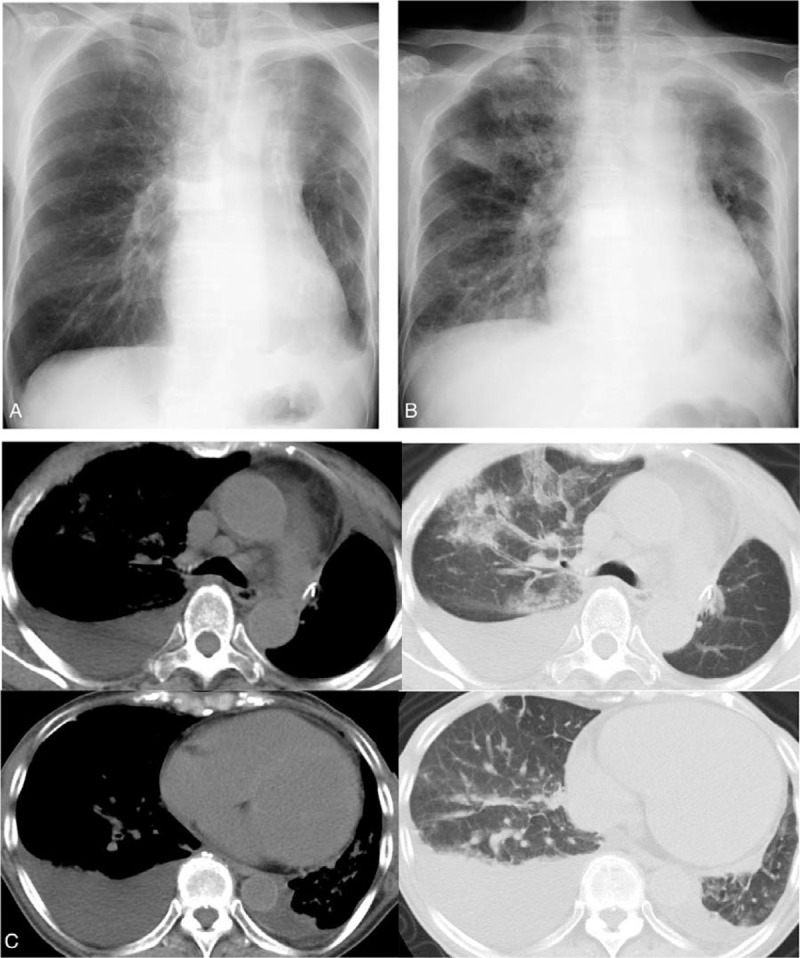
Chest radiography indicates an enlarged cardiac shadow and diffuse ground-glass opacities in both lung fields on admission (A). After osimertinib discontinuation, the chest radiograph displays an improvement in enlarged cardiac shadow (B). Chest CT exhibits cardiac enlargement, pleural effusion, and ground-glass opacities in both lung fields (C).
